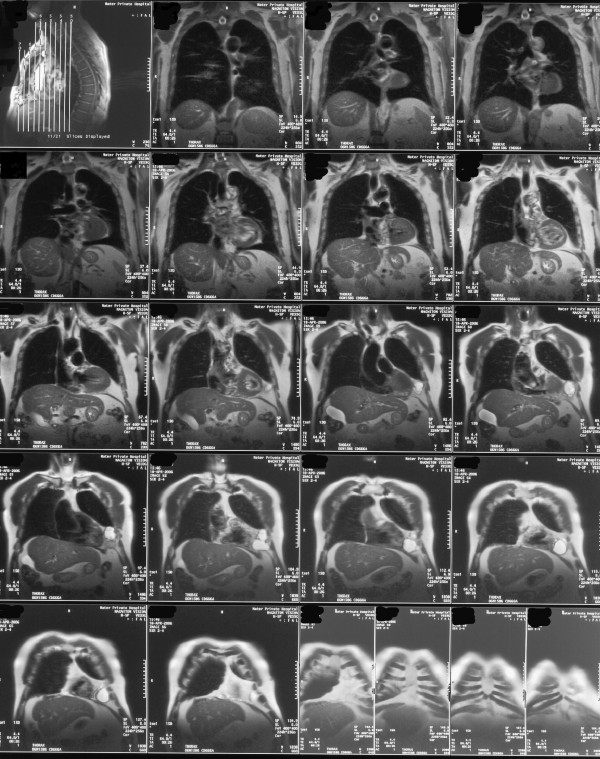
MRI Scan of the thorax showing the pericardial cyst.
